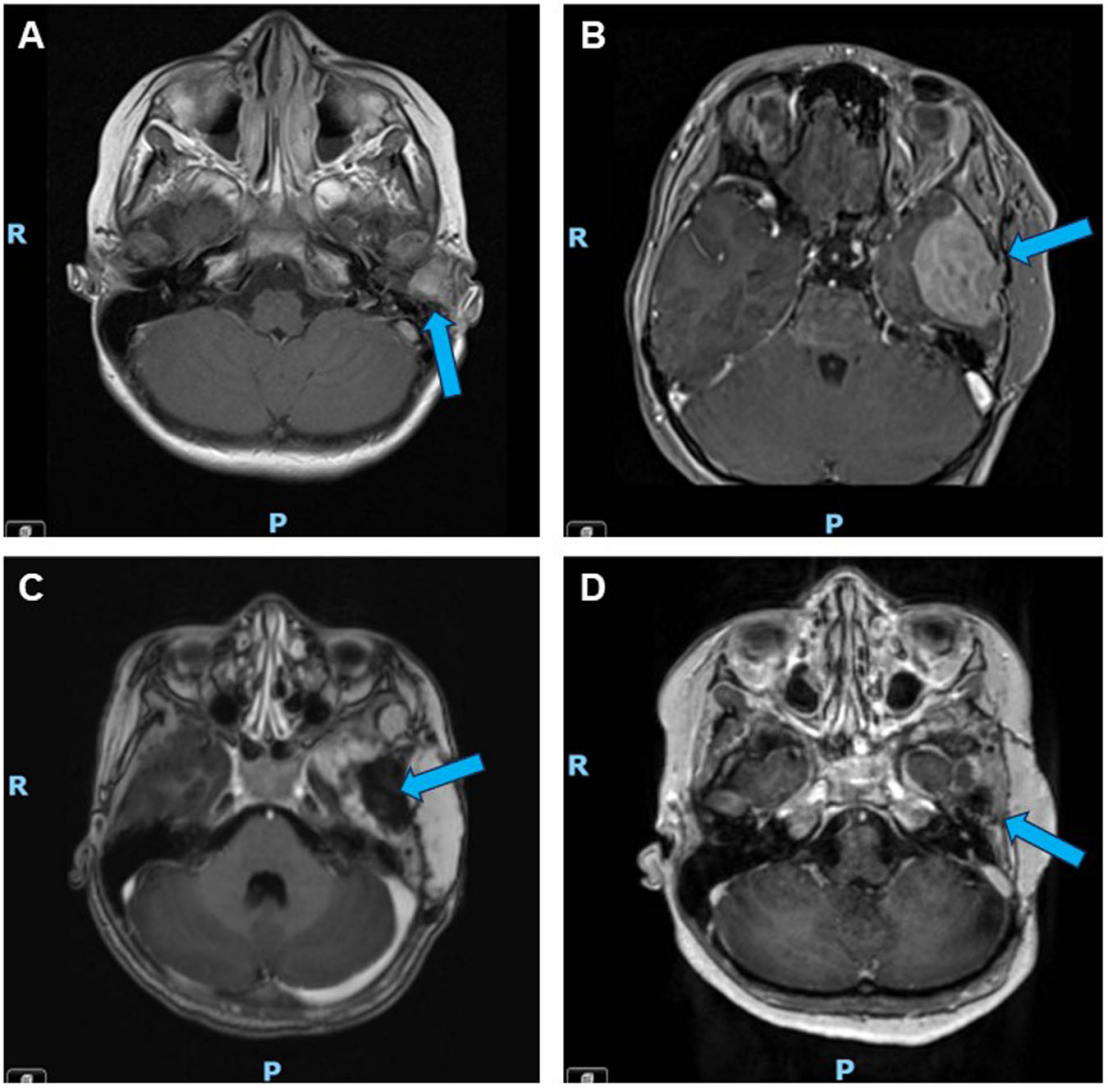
Axial T1 MRI images of the brain through the course of treatment. A First relapse; a 1.4 cm tumor was identified in the left external auditory canal three months after initial debulking surgery and prior to any systemic treatment. B Second relapse; after one year of adjuvant nivolumab, the patient experienced a second relapse when a new 4.0 cm mass was identified along the floor of the left middle cranial fossa with mass effect on the left temporal lobe. C Post-operative imaging; the patient underwent intracranial tumor resection after two cycles of neoadjuvant ipilimumab/ nivolumab. D Representative surveillance imaging while on tazemetostat; eighteen months post-operatively and fifteen months into tazemetostat therapy, the patient continued to demonstrate no evidence of relapsed disease. Blue arrows indicate the location of the tumor or resection site.
